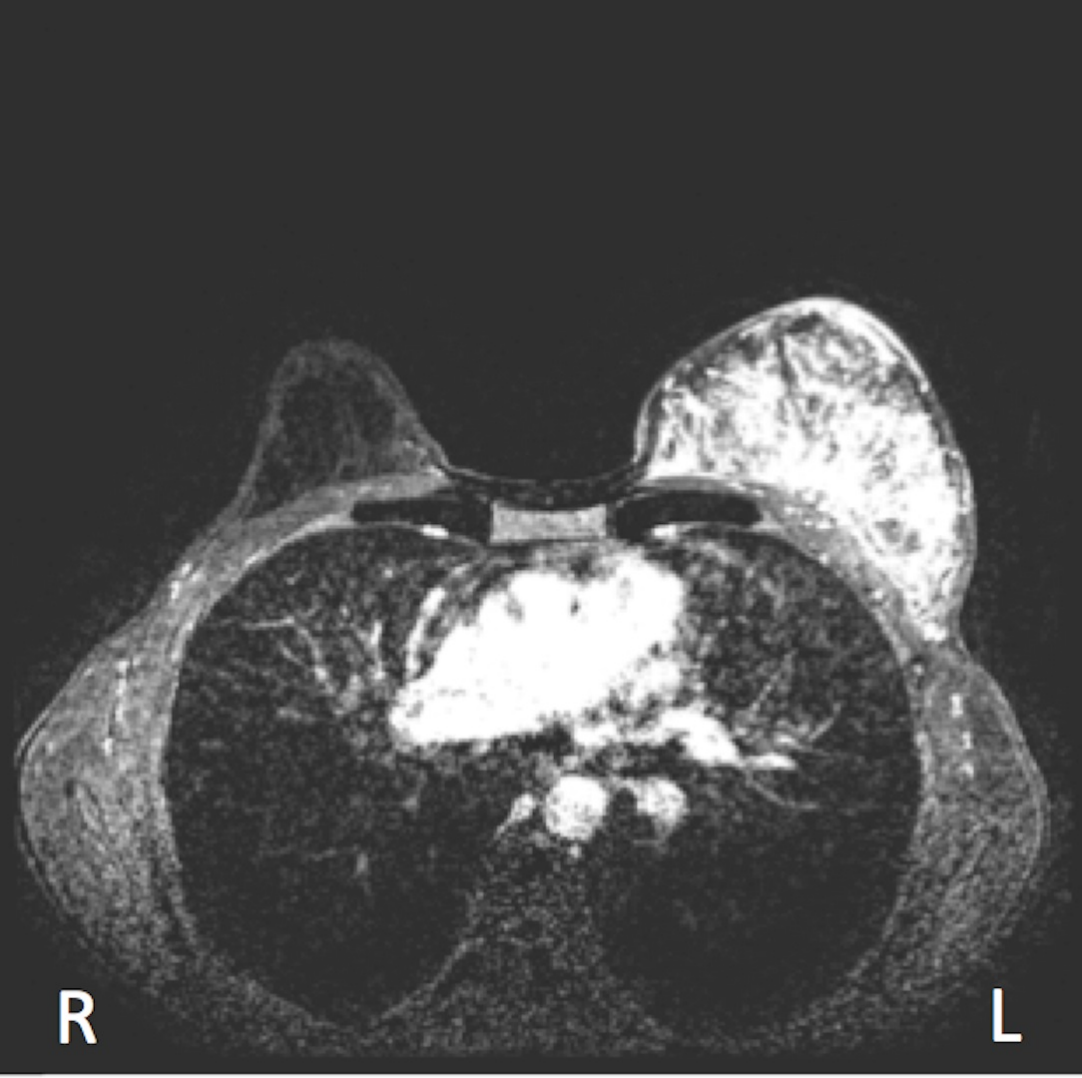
Magnetic resonance imaging showing an inflammatory response in the breast, soft tissues and skin after the first cycle of docetaxel and trastuzumab therapy
